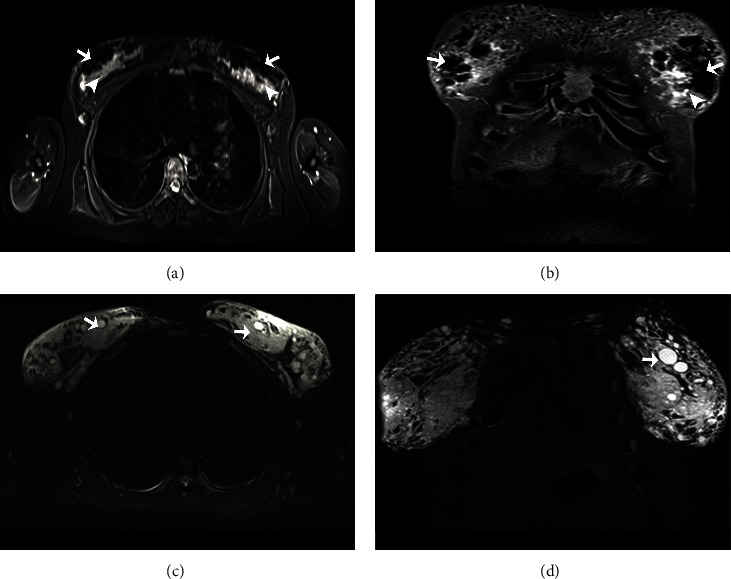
Magnetic resonance imaging STIR sequence showing different patterns in the breasts, in two patients, with the infiltration of silicone and other substances. Patient 1: it was used silicone, an approximate amount of 1250 ml. (a) Axial plane: there is infiltration by modelling material with a mixed and nodular pattern, with hypointense signal in the superficial portion (white arrows) and a diffuse pattern with hyperintense signal in the deep part of both breasts (white arrowheads); right axillary ganglion with modeling infiltration is also identified. (b) Coronal plane: the hypointense signal's globular pattern also affects the intermammary region's subcutaneous cellular tissue (white arrows). Patient 2: it was a mixture of mineral oil, car's oil, and silicone, with an approximate amount of 2000 ml. (c) Axial plane: a globular pattern of hyperintense signal is observed (white arrows), associated with diffuse hyperintensity changes due to diffuse infiltration in both breasts. (d) Coronal plane: globular pattern of hyperintense signal predominantly in the left breast (white arrow).
